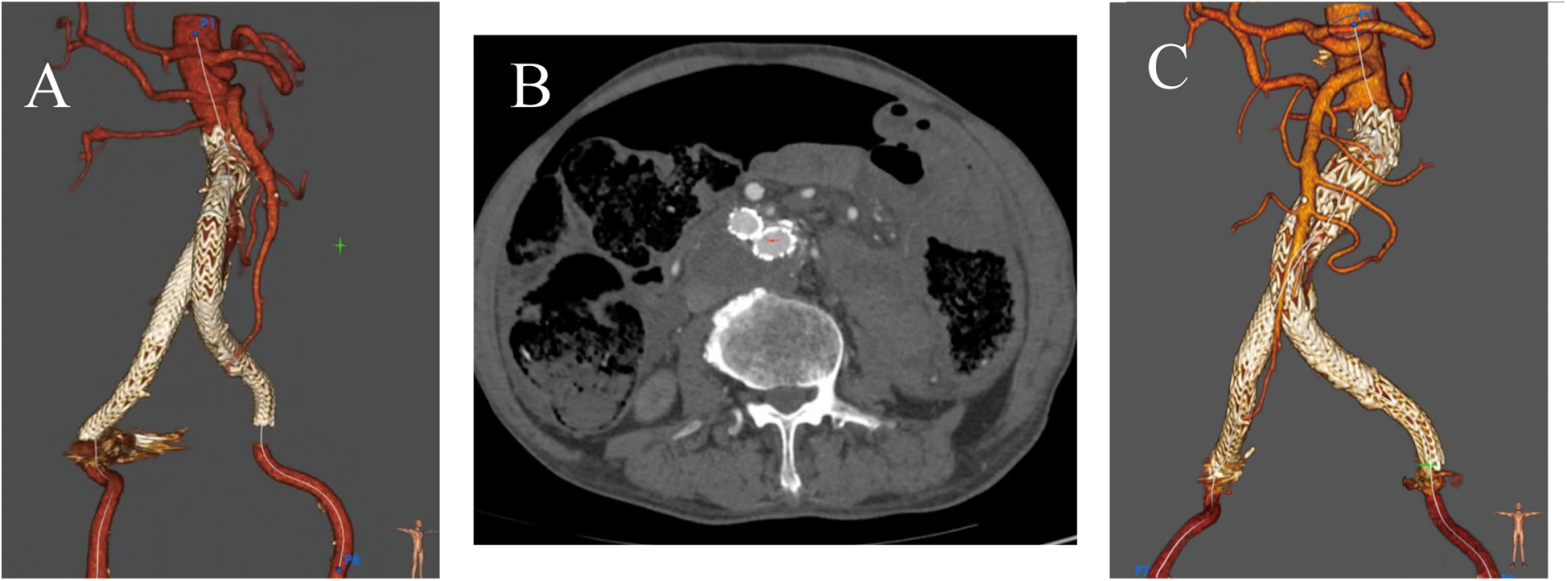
(A) Postoperative CTA showed no evidence of the fistula or the endoleaks. (B,C) At the 9-month follow-up, CTA showed no evidence of endoleak or fistula.
